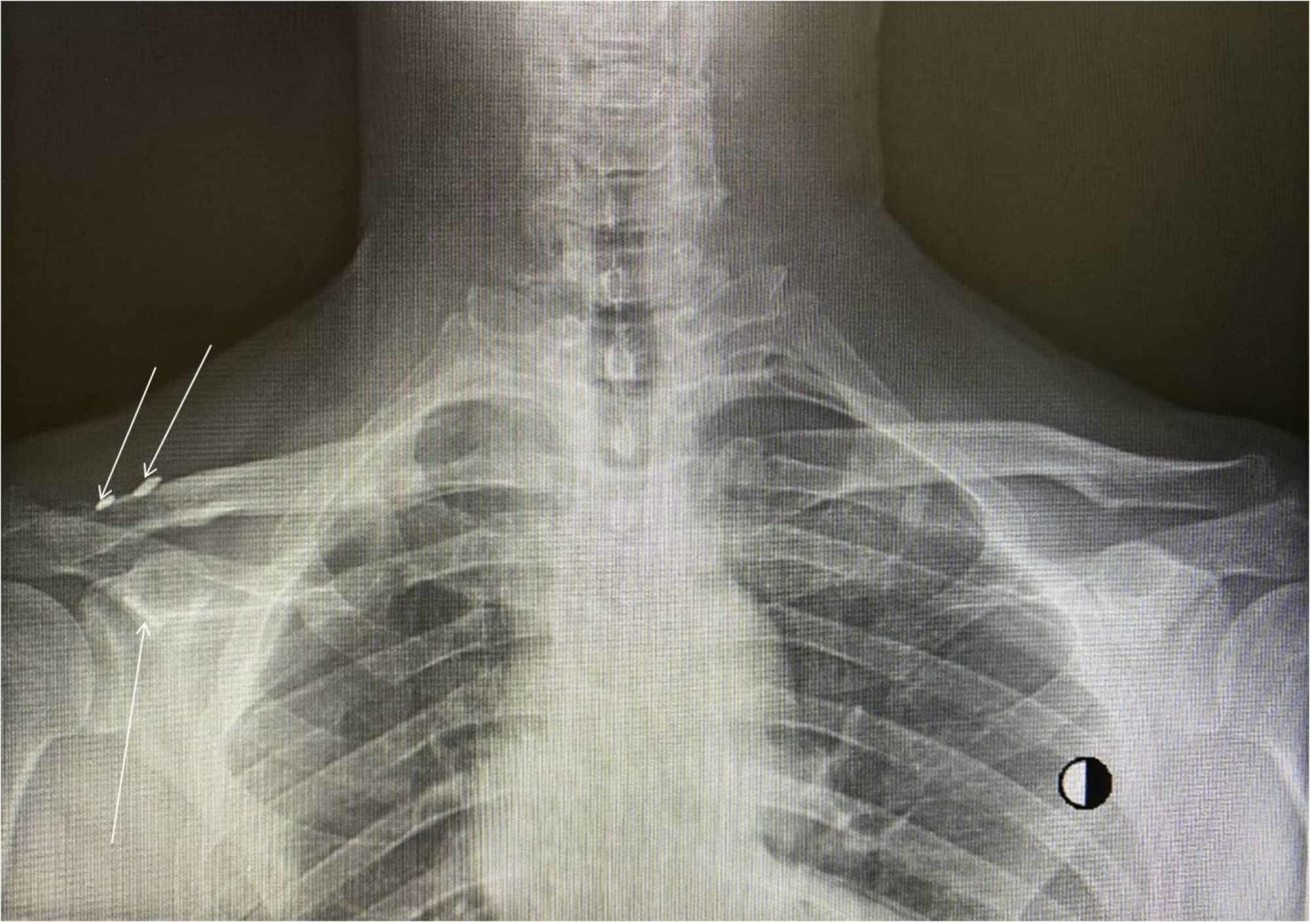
Radiograph demonstrating almost accurate reduction; however, functional results were excellent. The arrows point to the buttons of the "Y Button" configuration.
